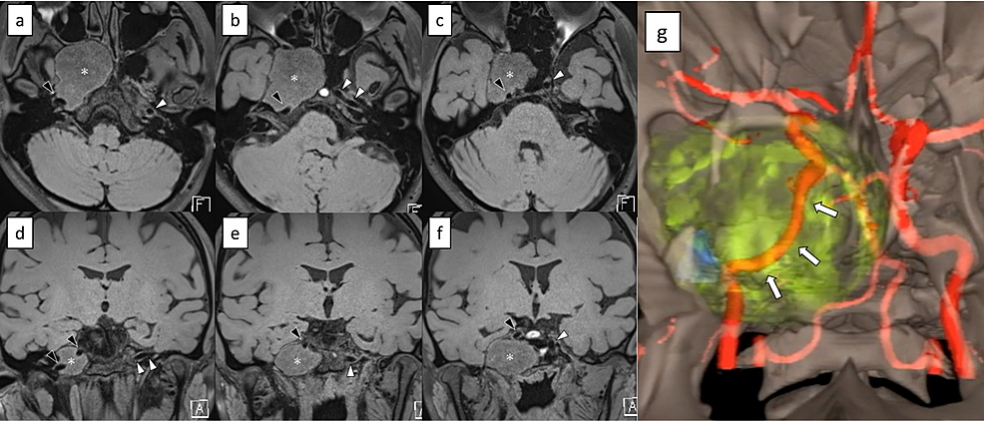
Preoperative assessment of the course of the internal carotid artery (a-f) Non-contrast MRI with DANTE-SPACE sequence. a) to c) are axial images (caudal to cephalad). d) to f) are coronal images (posterior to anterior). The internal carotid artery behind the tumor was appreciated by black blood image. g) Virtual image made from MRA and MRI using the surgical navigation system. Red: artery; yellow: tumor; blue: foramen ovale. The paraclival and petrous portion of the internal carotid artery on the right side was postero-laterally displaced by the tumor (arrows). Asterisk: tumor. Black arrowhead: internal carotid artery on the right side. White arrowhead: internal carotid artery on the left side.
